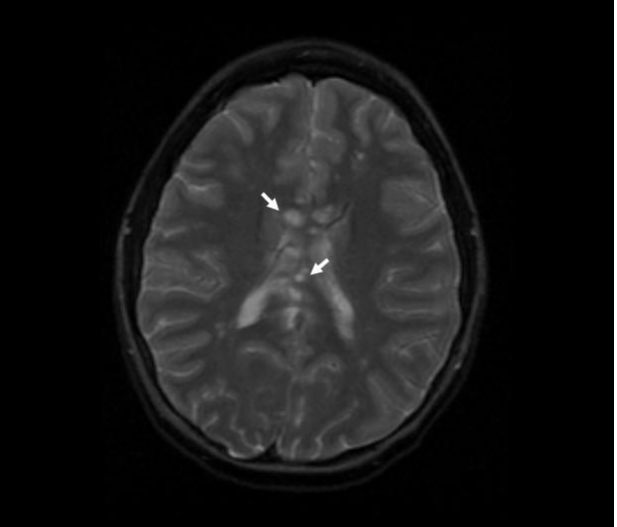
MRI brain. Axial T2 image showing multiple white matter lesions, especially in the corpus callosum. The arrows point to only two of several callosal lesions. Early in the disease, callosal lesions are usually best seen on thin section sagittal FLAIR and sagittal T1 images, with contrast.
